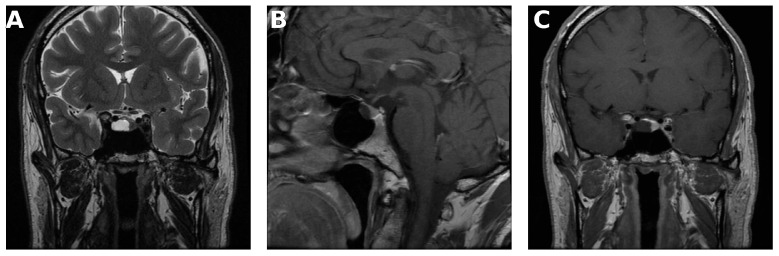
(A) Coronal T2-weighted image demonstrating thin internal septations within a cystic pituitary adenoma. (B) Contrast-enhanced sagittal T1-weighted image demonstrating anterior localization of the lesion. (C) Contrast-enhanced coronal T1-weighted image demonstrating paramedian localization.
